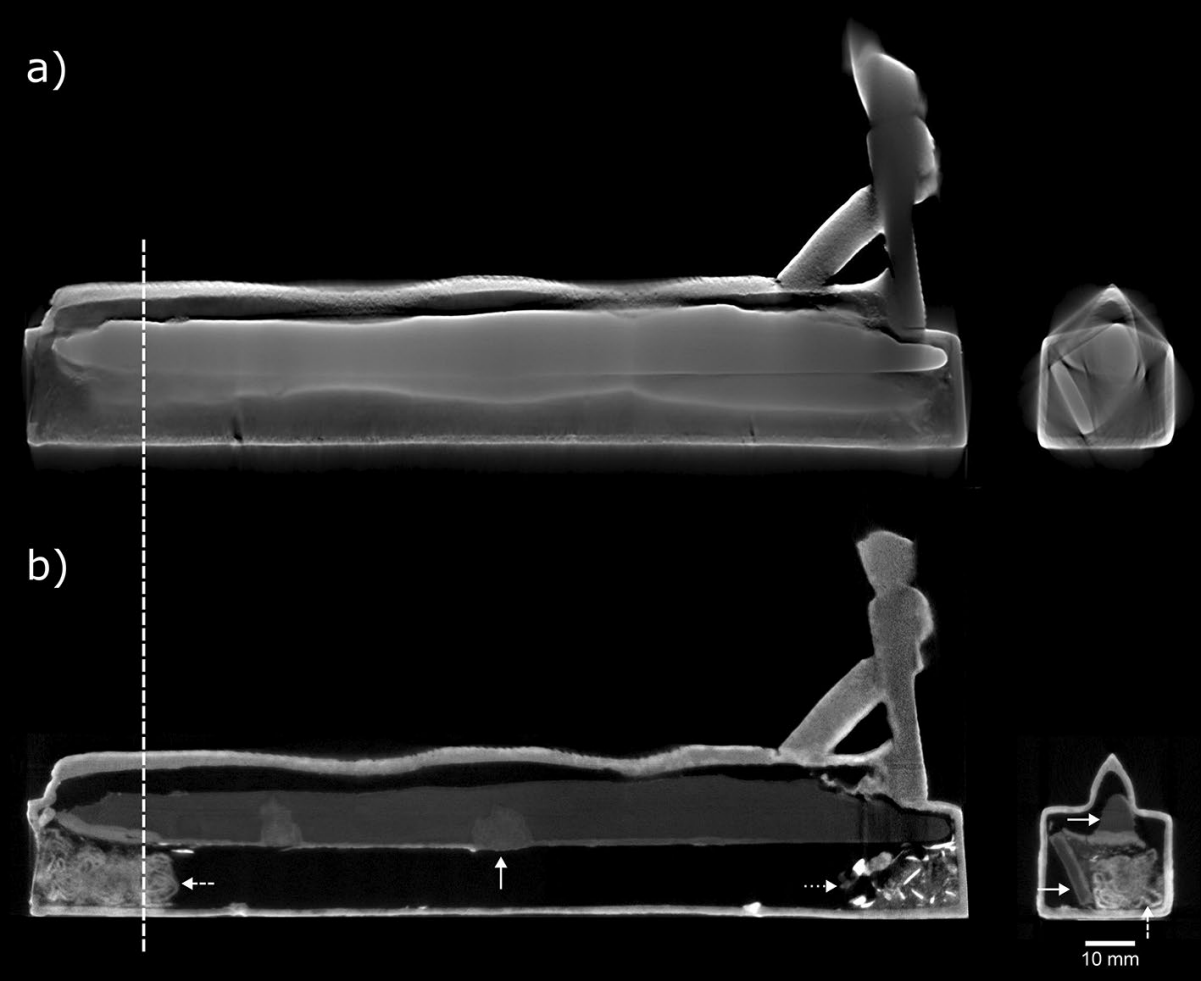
EA71428 (a) X-ray and (b) neutron CT slices (side-view and front-view). Front-view slices taken at the position marked by the dashed line. The neutron CT images are averaged over two adjacent planes (giving a 0.110 mm slice thickness), to approximately match the X-ray CT data (0.108 mm slice thickness). Solid arrows: lead pieces (in the neutron CT side-view, the arrow is centred on a region of deep lead corrosion in the upper lead piece); dashed arrows: textile in plug; dotted arrow: loose fragments. X-ray CT data were acquired during the previous study^9^.
